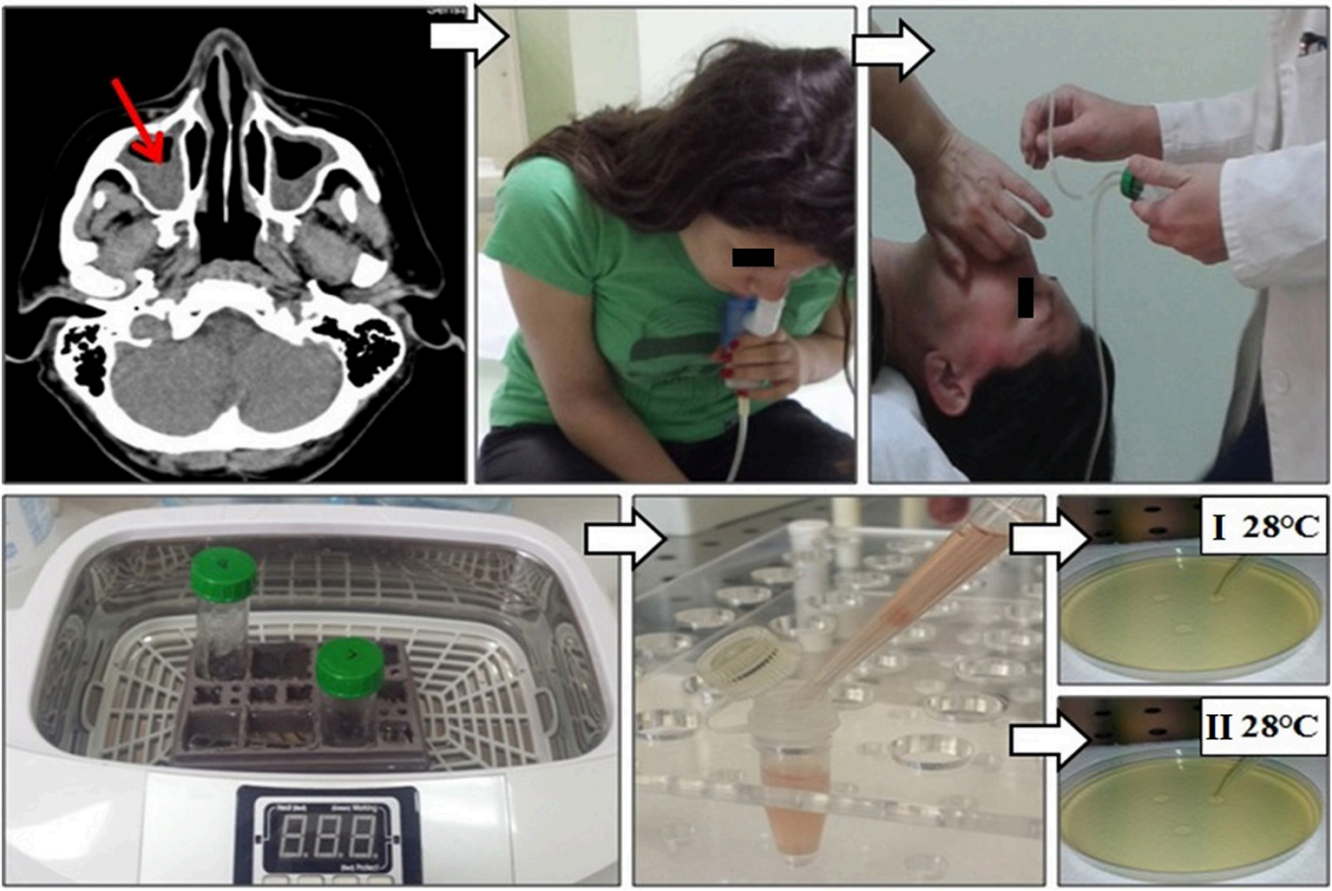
Sinonasal aspirate processing algorithm: (i) CT imaging of paranasal sinuses of patients with ABPA revealed CRS; (ii) each patient did the inhalation by PARI-SINUS nebulizer with hypertonic-NaCl solution for 10 min in the quadruped position; (iii) after the inhalation mucin was collected from the sinuses by aspiration with mucus extractor in a supine position with head tilted backward; (iv) the sinonasal aspirate was additionally processed in ultrasound cleaner aimed to prepare single-cell solution, and (v) afterwards single-cell solution was cultured at Sabouraud dextrose agar at 28°C for 7 days. Fungi were regarded as causative when the same fungi was isolated from sinonasal aspirate repeatedly.
